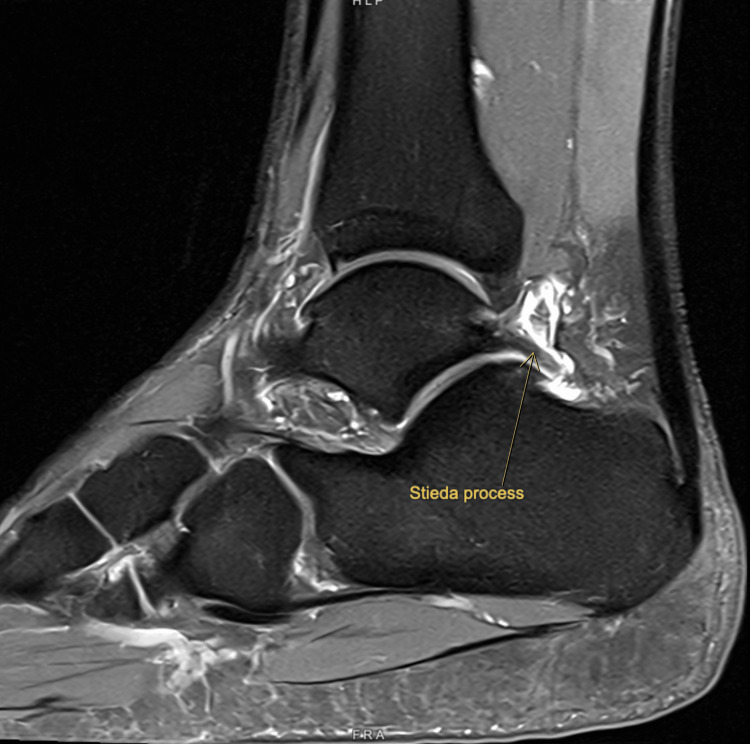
Sagittal T2-weighted MRI of the left ankle Sagittal fat-suppressed T2-weighted MRI of the left ankle demonstrating features of posterior ankle impingement, including an elongated Stieda process with associated bone marrow edema.
